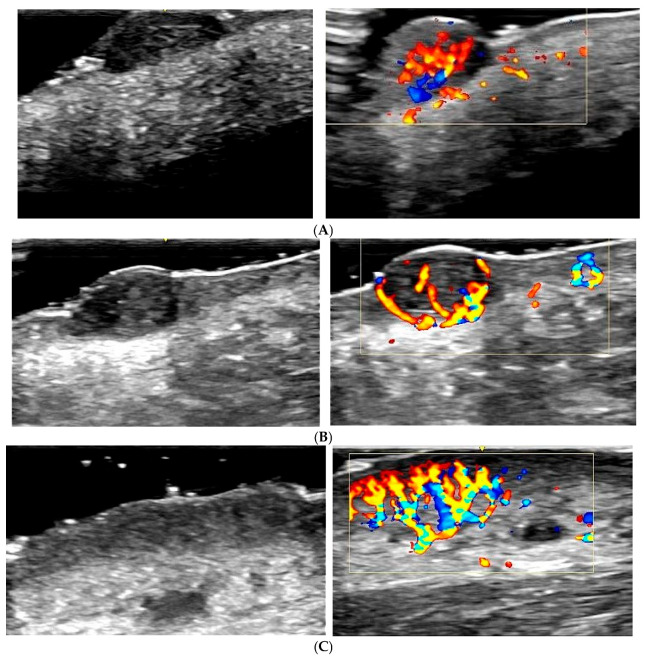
Colour Doppler ultrasound features of malignant cutaneous lesions. (A) *Melanoma:* Intense and disorganized vascular flow with irregular, chaotic patterns throughout the lesion. These findings are indicative of neovascularization and aggressive biological behaviour. (B) *Basal cell carcinoma (BCC):* Prominent peripheral vascularization with dilated vessels surrounding the lesion. Although less chaotic than melanoma, the increased vascular density supports malignancy. (C) *Squamous cell carcinoma (SCC):* High-velocity, intense vascular flow both centrally and peripherally. The combined pattern of disorganized vascularity and signal intensity is strongly suggestive of a malignant tumour.
